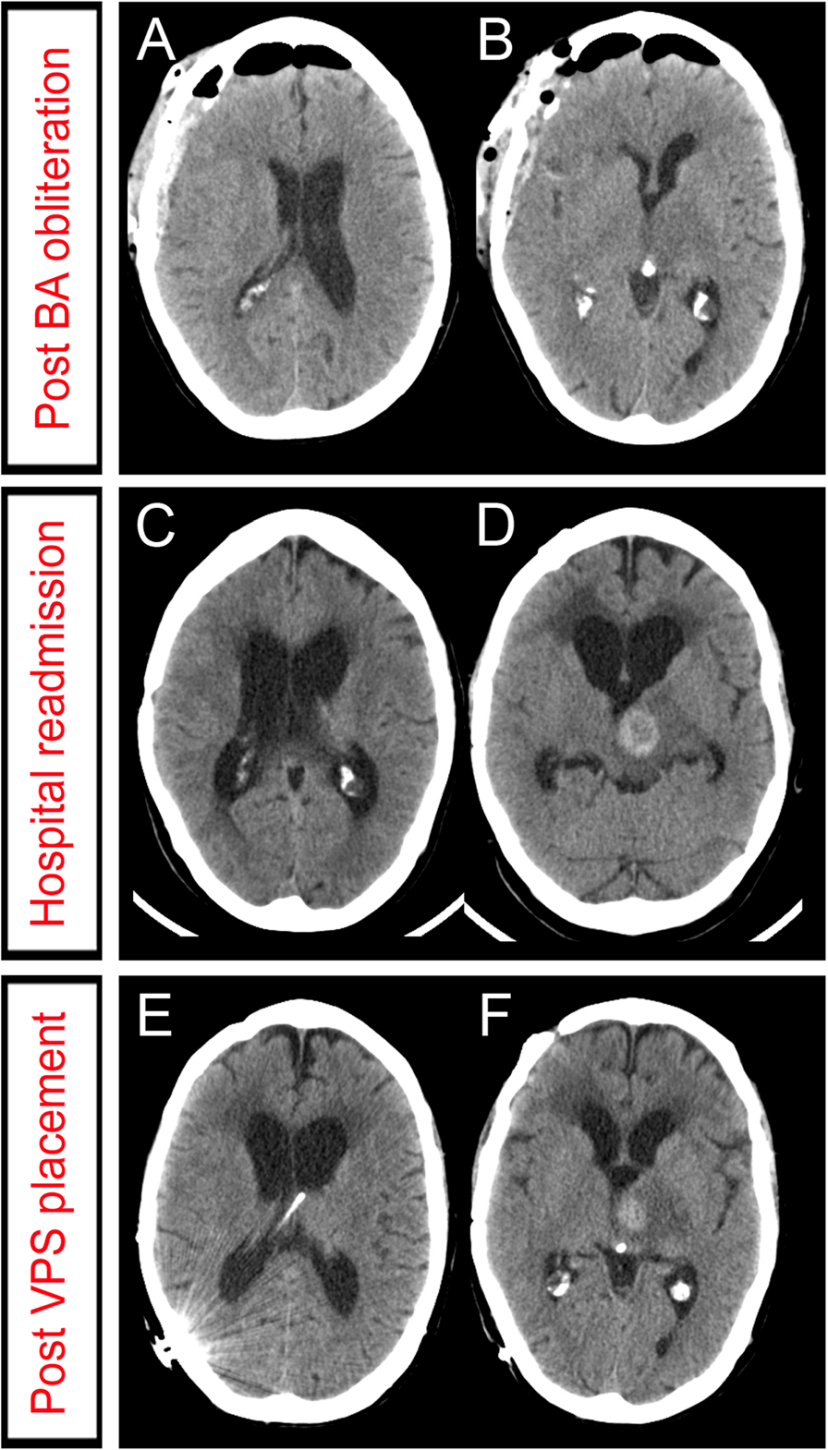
Follow up computed tomography (CT) detected a marked increase in the size of the supratentorial ventricular system together with clinical symptoms suggestive of hydrocephalus. a-b: Post-surgical changes secondary to a pterional craniotomy and a transsylvian approach, with pneumocephalus and blood remnants in the surgical site. c-d: Plain CT performed at the emergency department 3 months after surgical basilar artery occlusion. The supratentorial ventricular system was markedly increased in size compared to the previous study. We also noticed low attenuation periventricular changes around the lateral ventricles (LVs), thus suggesting transependymal edema. e-f: A right parieto-occipital ventriculoperitoneal shunt was placed, with the proximal catheter arising from the occipital horn of the right LV. Notice the decrease in the size of the supratentorial ventricular system in comparison with the previous study
